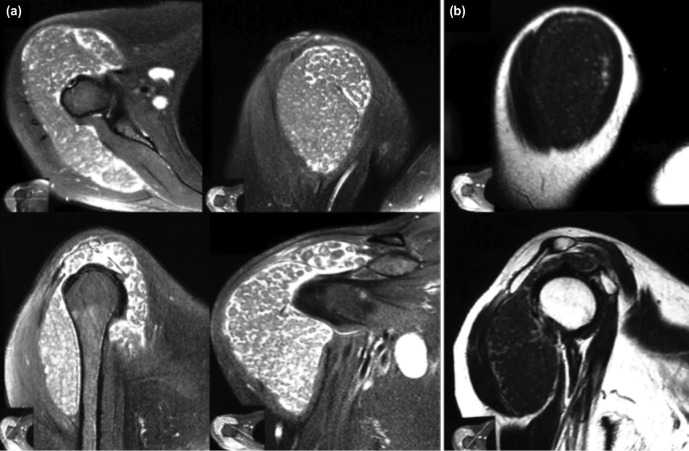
(a) MRI of right shoulder axial, coronal and sagittal T1 weighted images showing multiple iso-intense rice bodies. (b) MRI of right shoulder T2 weighted images showing hypo-intense rice bodies.
